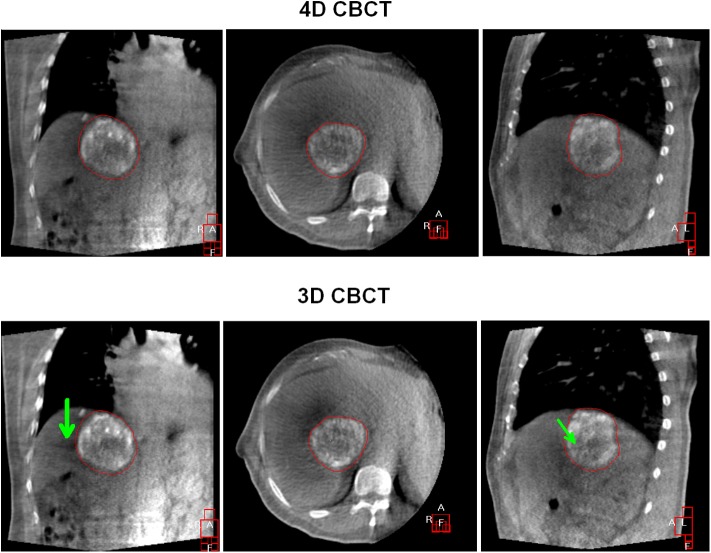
Registration results are highly similar between 3D– and 4D–CBCT (displayed in same window / level). Effect of motion artifact is more pronounced on the 3D–CBCT images (bottom) than on the time–weighted average 4D–CBCT images (top), as indicated by the green arrows. The lipiodol contrast, however, is visualized brighter on 3D–CBCT than on 4D–CBCT due to a tenfold increase of projection data.
